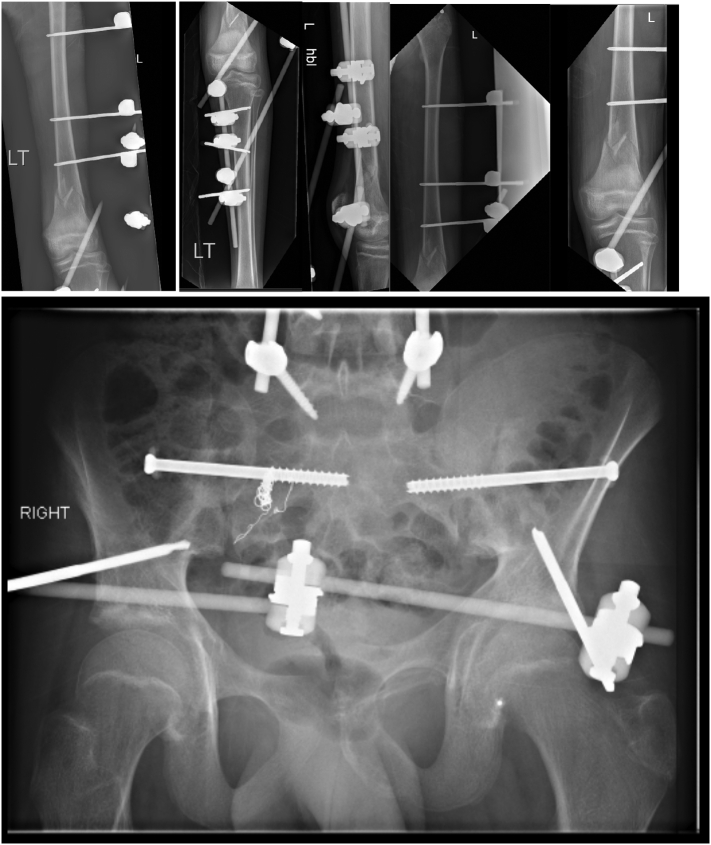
Post-operative radiograph (eight weeks) of the pelvis and left femur, shows posterior stabilisation from L1 to L5, external fixation in situ of the pelvis and left femur.
